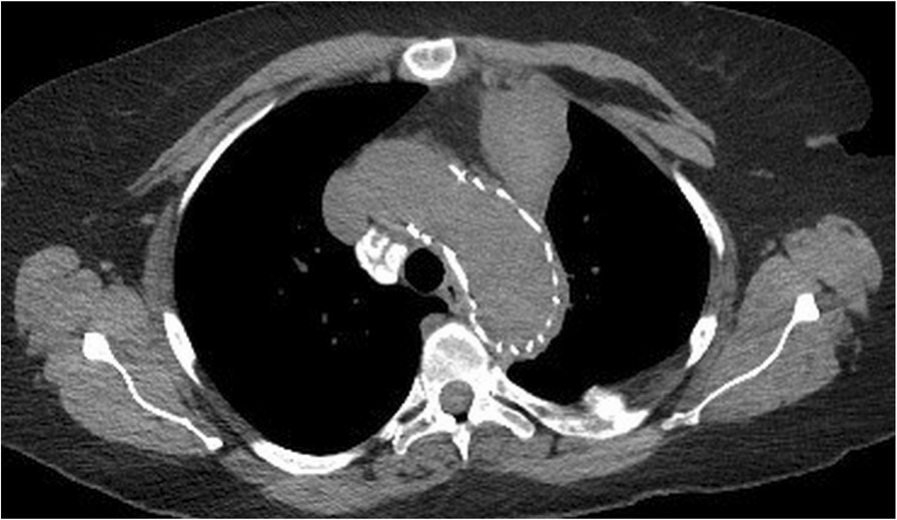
Follow up CTA (axial view) one month after TEVAR showing an aortic stent graft and resolution of intramural hematoma
